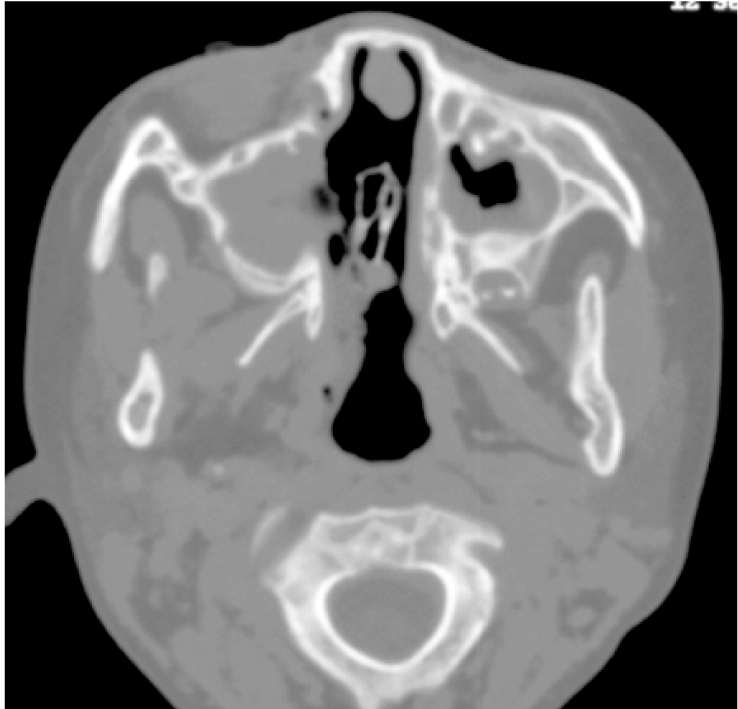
Fungal sinusitis of a four-year-old girl with myeloid leukaemia. CT of the sinuses revealed opacification of the maxillary sinuses with bone destruction of the medial wall. Mucor species was isolated from maxillary sinus washout.
